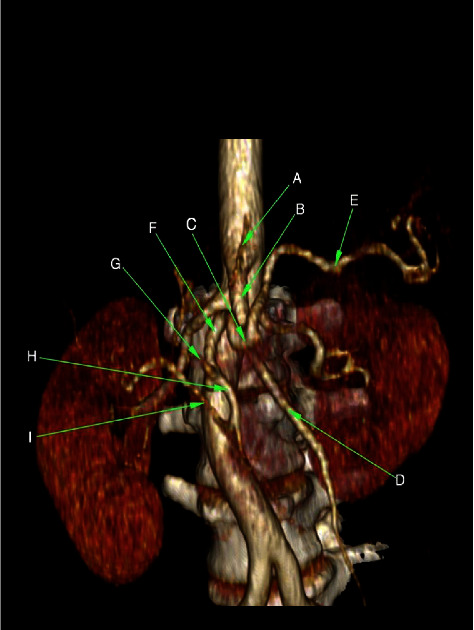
Three-dimensional volume rendering CT image demonstrating a hepato-spleno-mesenteric trunk. *A* = left gastric artery, *B* = celiacomesenteric trunk, *C* = celiac trunk, *D* = superior mesenteric artery, *E* = splenic artery, *F* = common hepatic artery, *G* = left hepatic artery, *H* = gastroduodenal artery, and I = right hepatic artery (reproduced with permission from Johnson PB, et al. Vascular Supply to the Liver: Report of a Rare Arterial Variant. Case Rep Radiology. 2013.969327:1-3. DOI: 10.1155/2013/969327 [2]).
